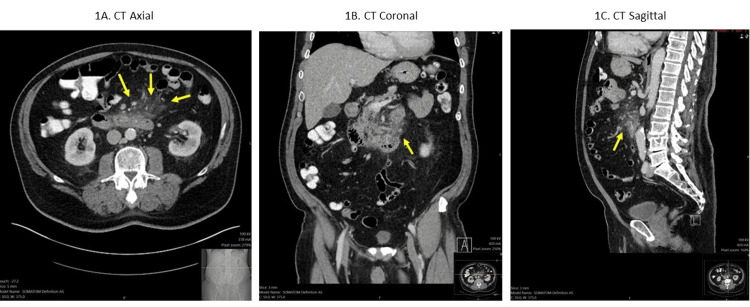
Axial (1A), coronal (1B), and sagittal (1C) CT scans of the abdomen and pelvis with IV contrast showing stranding and inflammation around the duodenum and pancreas, read as pancreatitis/duodenitis. Note the inflammation obscuring the visualization of the grill bristle brush in the third portion of the duodenum in Figures 1A and 1B, making delineation of the foreign body by CT challenging.
